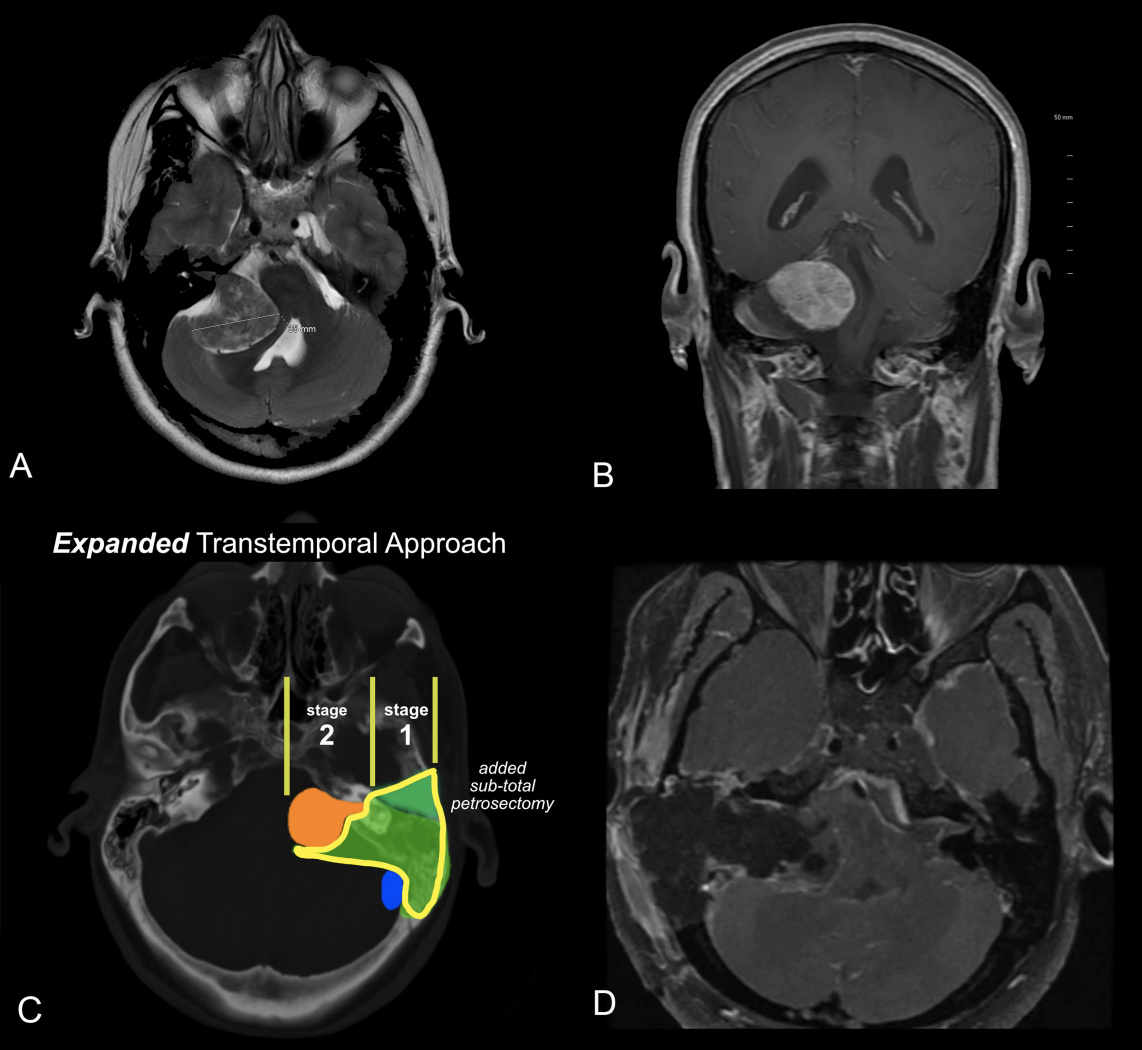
(A, B) MRI of a patient with a very large acoustic neuroma. (A) T2 axial MRI. (B) T1 C+ coronal MRI. (C) Expanded Transtemporal Approach. The green segment, lower portion, is the same as the translabyrinthine resection. The upper green segment is the additional bone removed from the subtotal petrosectomy. The lateral bone removal can easily be performed as a separate stage from the tumor removal to optimize safety and outcomes. (D) Post-operative MRI revealing gross total removal by the expanded transtemporal approach.
